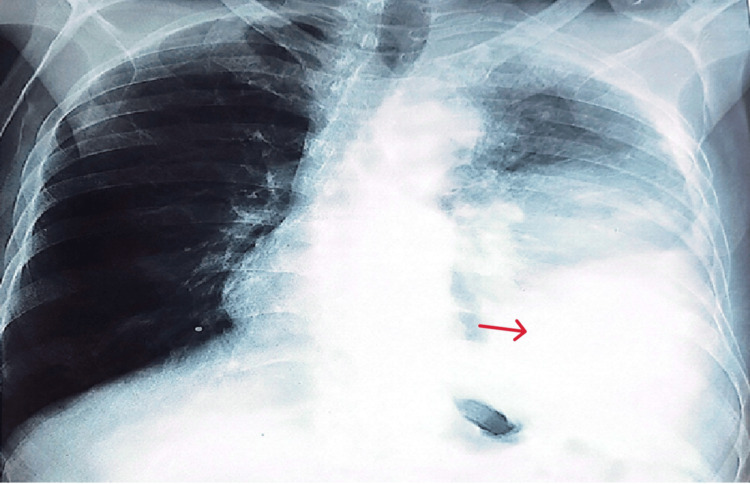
Chest X-ray showing a left basal loculated pleural effusion with an overlying heterogeneous retractile opacity. The red arrow indicates pleural effusion.
